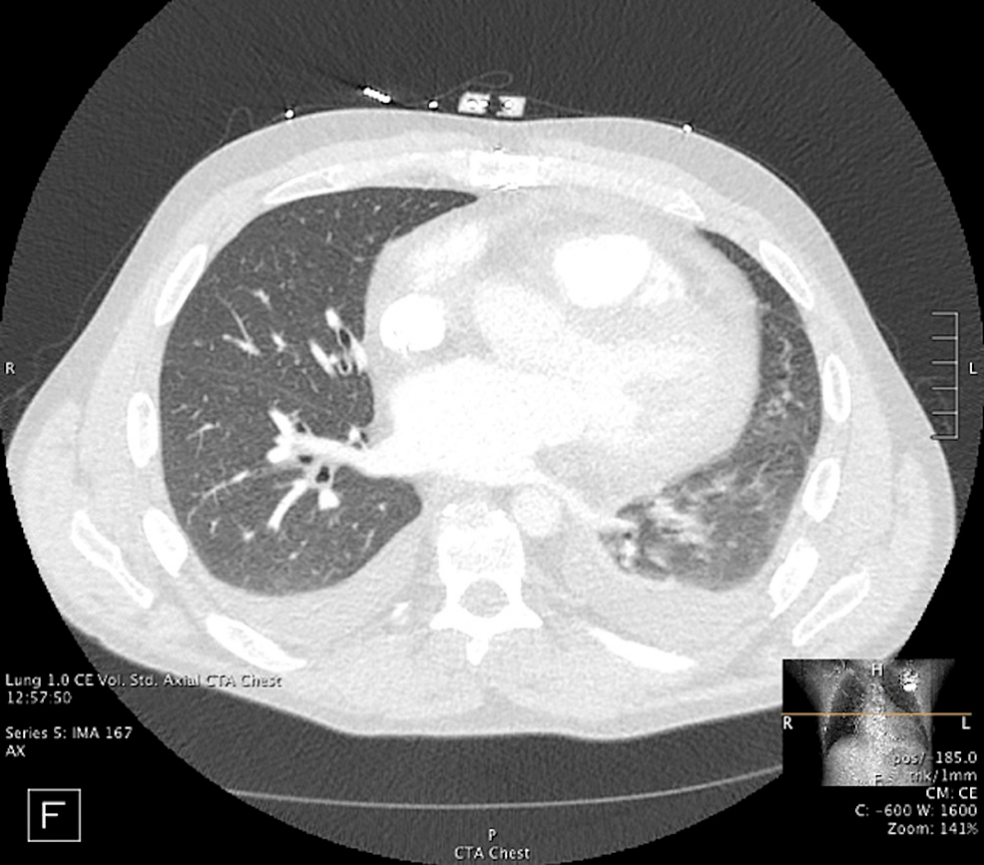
CTA showing evidence of mild pulmonary edema and bilateral pleural effusions CTA: computed tomography angiography
